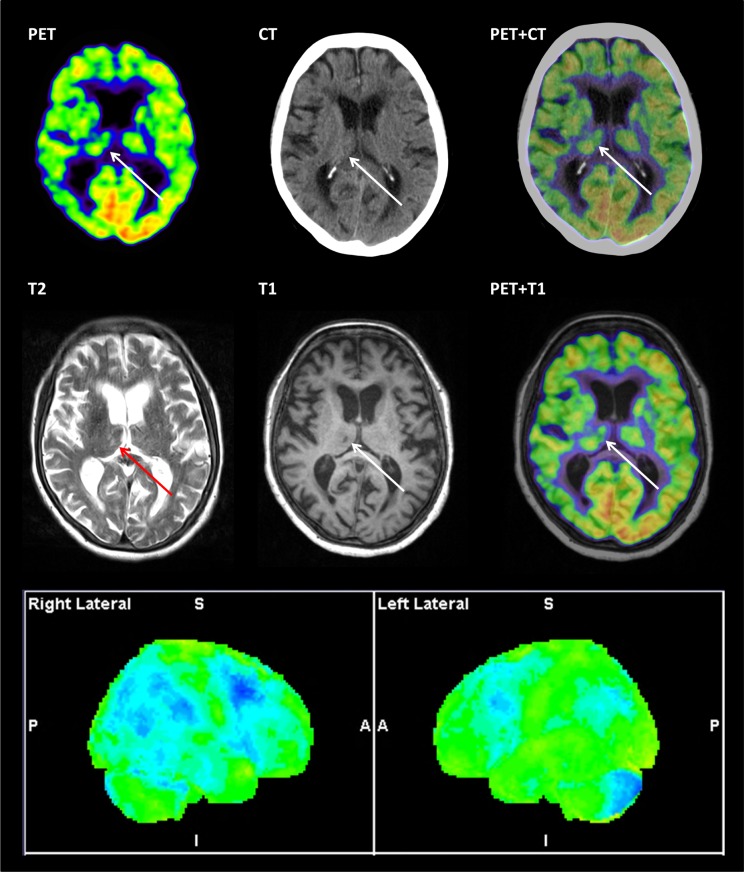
Major clinical impact. Upper rows show corresponding axial slices from CT, T2 and T1 MRI, and fused PET+CT and PET+T1. The two scans were performed 7 days apart. MRI shows a lacunar infarct in the right thalamus not visible on CT. Both MRI and CT were scored as Fazekas 2. Fused FDG PET and T1 MRI show a small metabolic defect in the right thalamic infarct (arrows). Statistical surface projections (lower row) show frontal and parietotemporal hypometabolism more pronounced in the right cerebral hemisphere and also lower uptake in the left cerebellar hemisphere. PET classification changed from neurodegenerative + vascular disease (white matter lesions) to predominantly vascular disease (white matter lesions + strategic infarct). Clinical diagnosis was changed from neurodegenerative to mixed and the finding was considered to constitute major impact (indication for platelet inhibitory drug).
